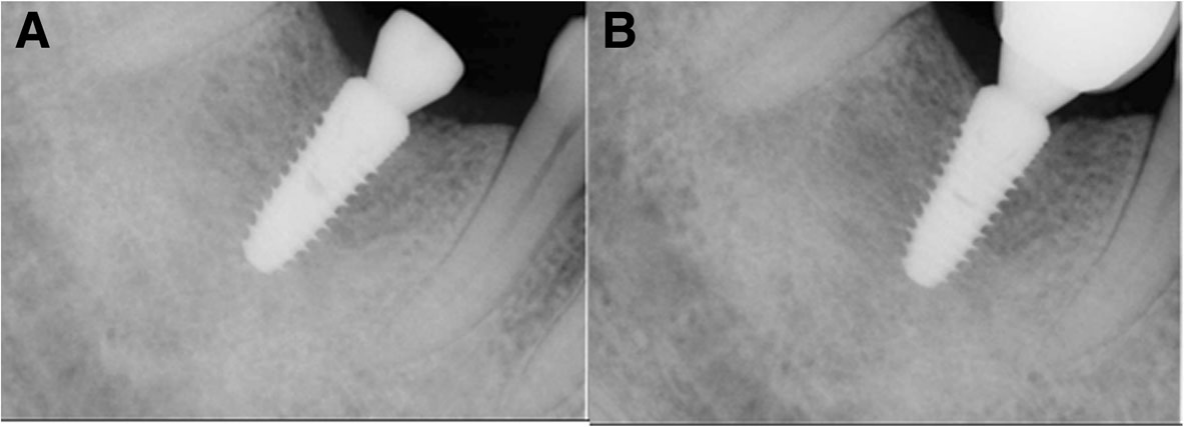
M3, periapical radiograph, a #46i, second operation, b #46i, prosthesis delivery
